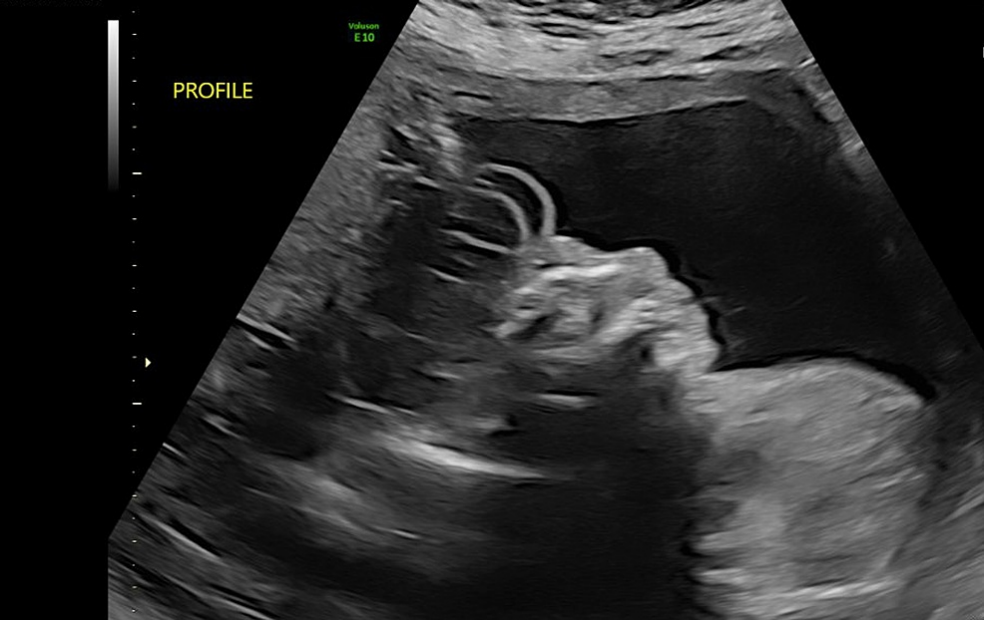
Ultrasound at 23 weeks noting: “acalvaria with an absence of the parietal, temporal, and occipital bones but with skull base and facial bones intact; disorganized brain tissue is seen floating in the amniotic fluid.”
